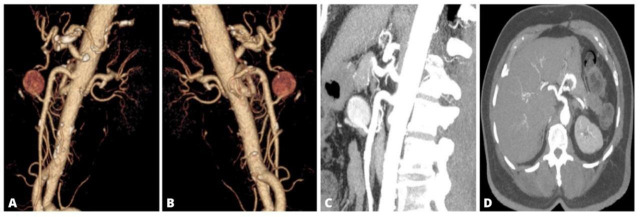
(A) Left anterior oblique volumetric angiotomographic reconstruction showing a large inferior pancreaticoduodenal artery aneurysm; (B) Right anterior oblique volumetric angiotomographic reconstruction showing communications with the celiac trunk (CT) via the gastroduodenal artery and with the superior mesenteric artery via the inferior pancreaticoduodenal artery; (C) Axial angiotomographic slice showing the ostial plaque in the CT; (D) Sagittal angiotomographic slice showing the ostial plaque in the CT causing approximately 20% stenosis.
